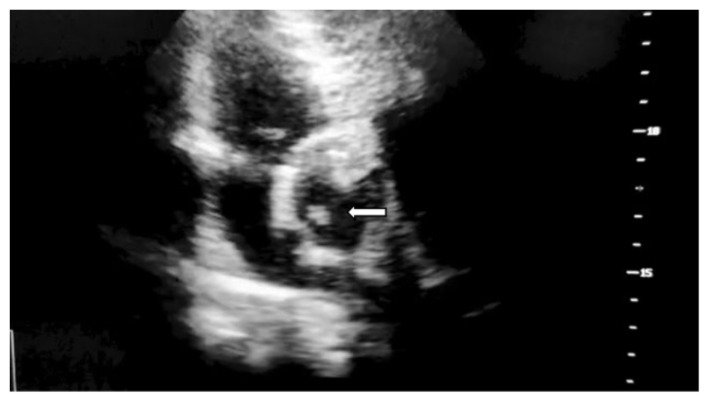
Transthoracic echocardiography hyperechogenic, serpentiform structure measuring 9.9 mm in maximum length, adherent to the aortic surface of the bioprosthetic valve at the fixation site with preserved mobility, marked with white arrow.
